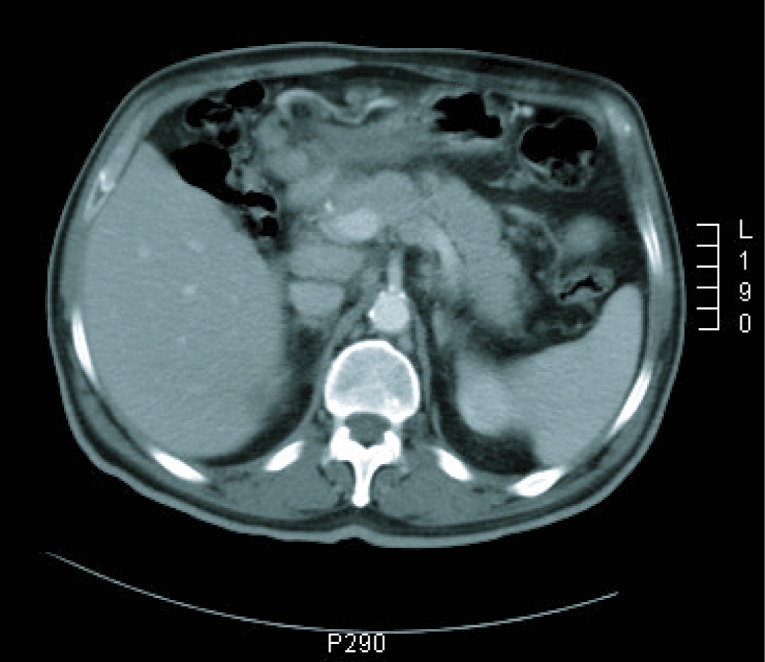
CT imaging at diagnosis.
